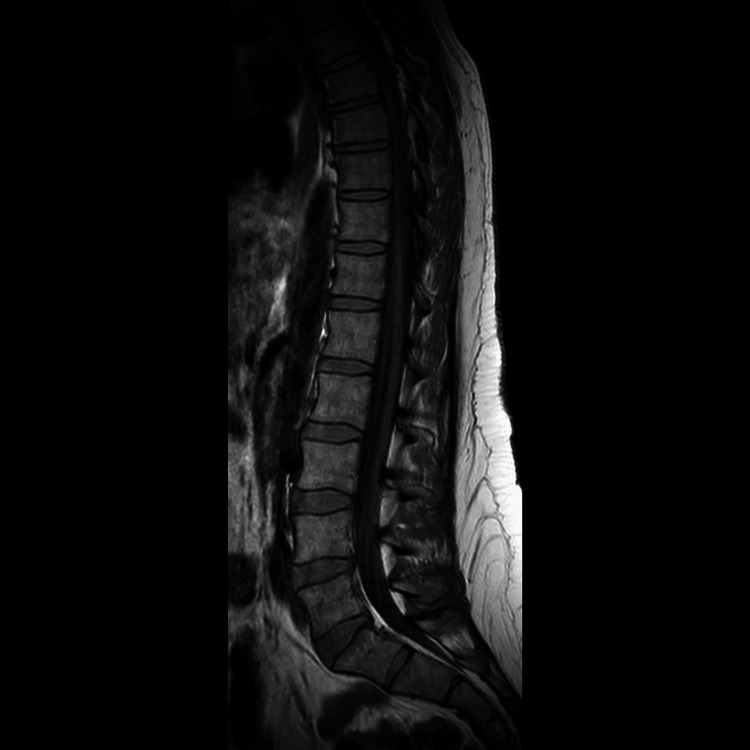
MRI of the thoraco-lumbar spine: normal with no signs of metastasis..
